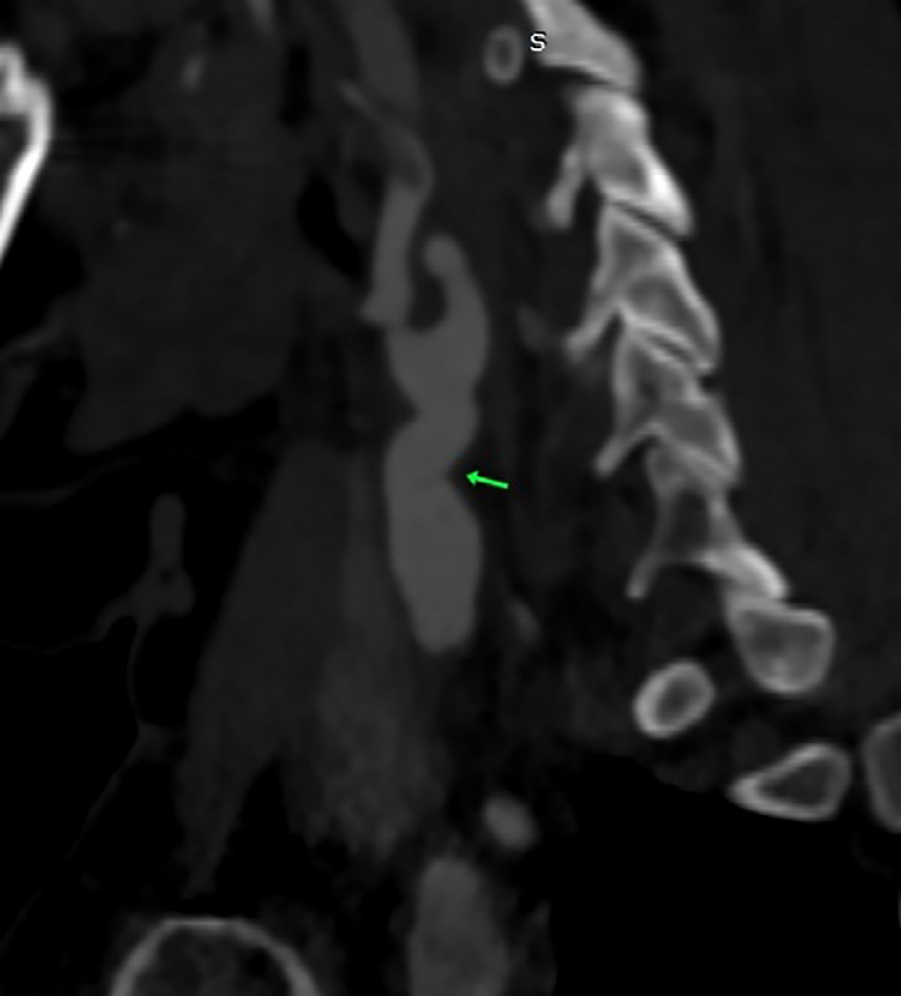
CTA showed a transverse, low density strip (arrow), causing luman stenosis, with proximal part aneurysmal enlargement on lower part of right CCA.
